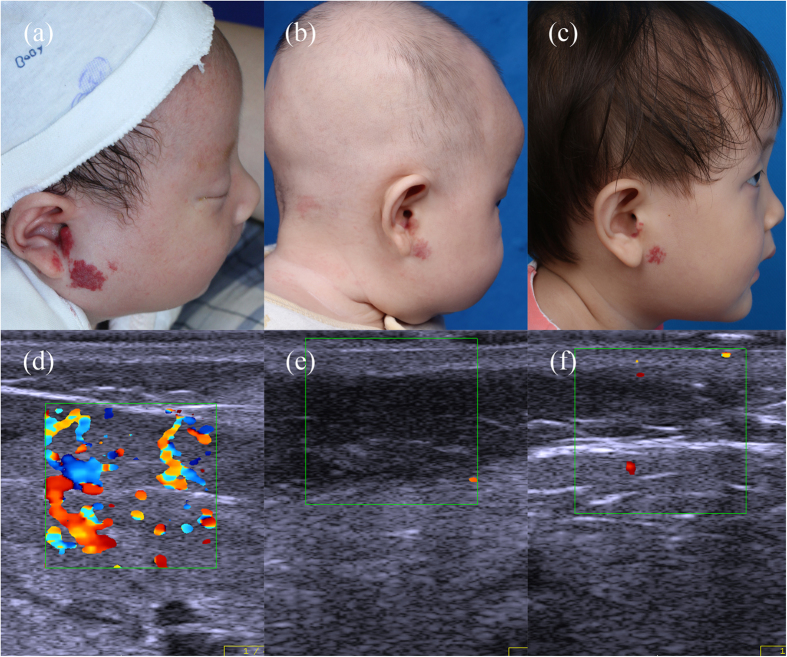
A typical complete regression case. (a) Before treatment, a 6-week-old girl presented with a mixed hemangioma in her right parotid region. (b) After 3 months of propranolol treatment, no obvious lesion remained. Propranolol was stopped gradually over 2 weeks. (c) The lesion was stable 23 months after propranolol was stopped. (d,e,f) Corresponding color Doppler ultrasound images of (a,b and c).
